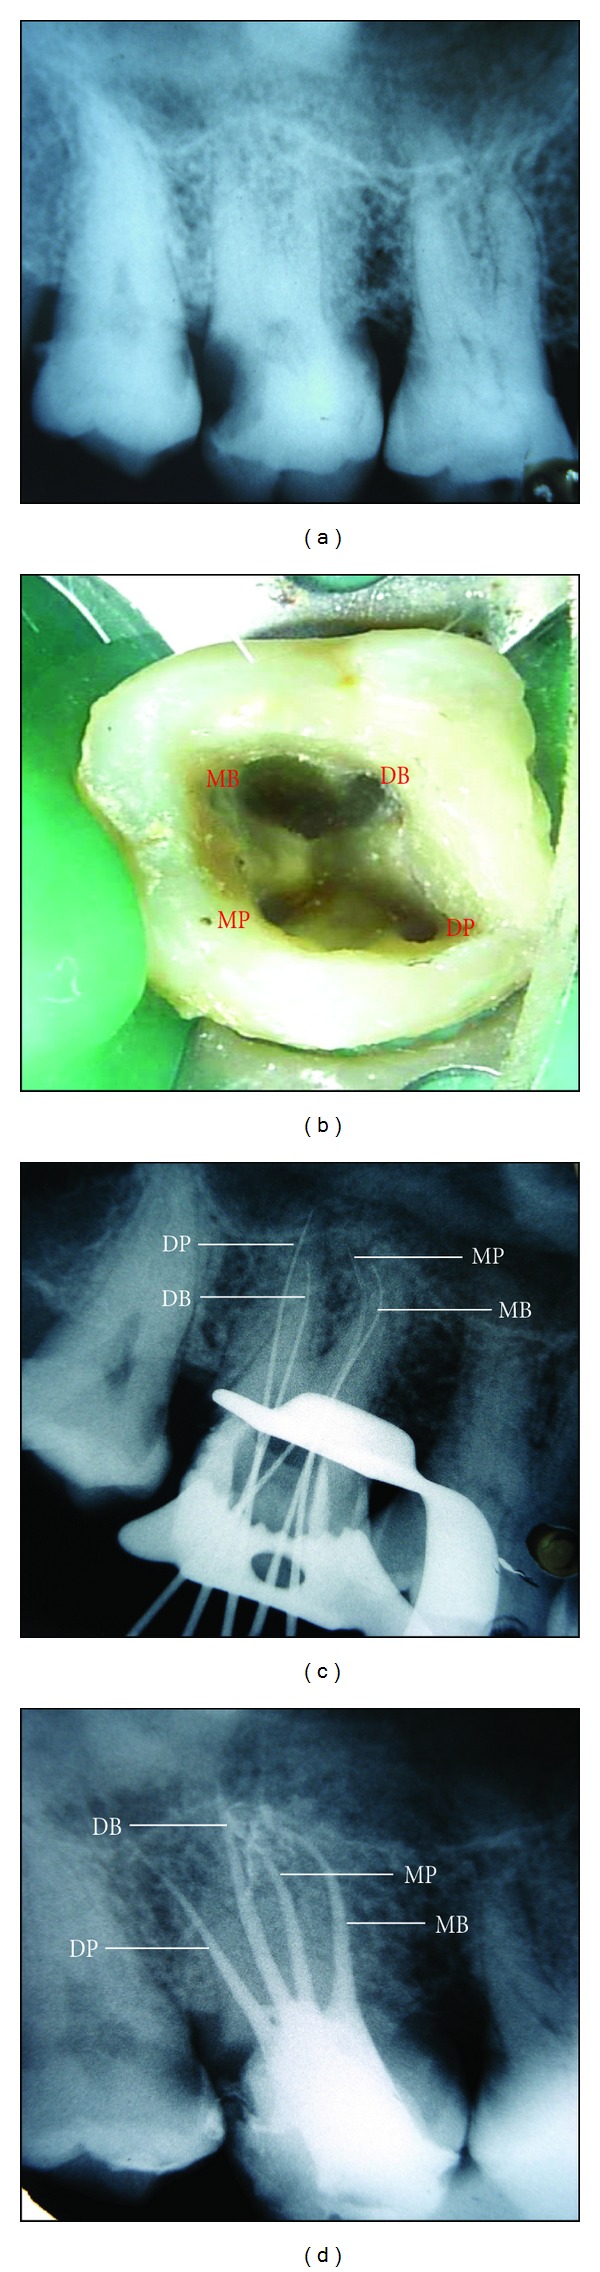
(a) Preoperative radiograph of the maxillary right second molar. (b) Access cavity revealing four distinct orifices. (c) Radiograph showing the working length of all four roots. (d) Postoperative radiograph of the obturated maxillary second molar.
